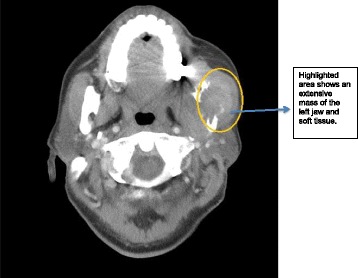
showing a contrast enhanced axial CT of the neck. Highlighted area shows an extensive mass of the left jaw and soft tissue
